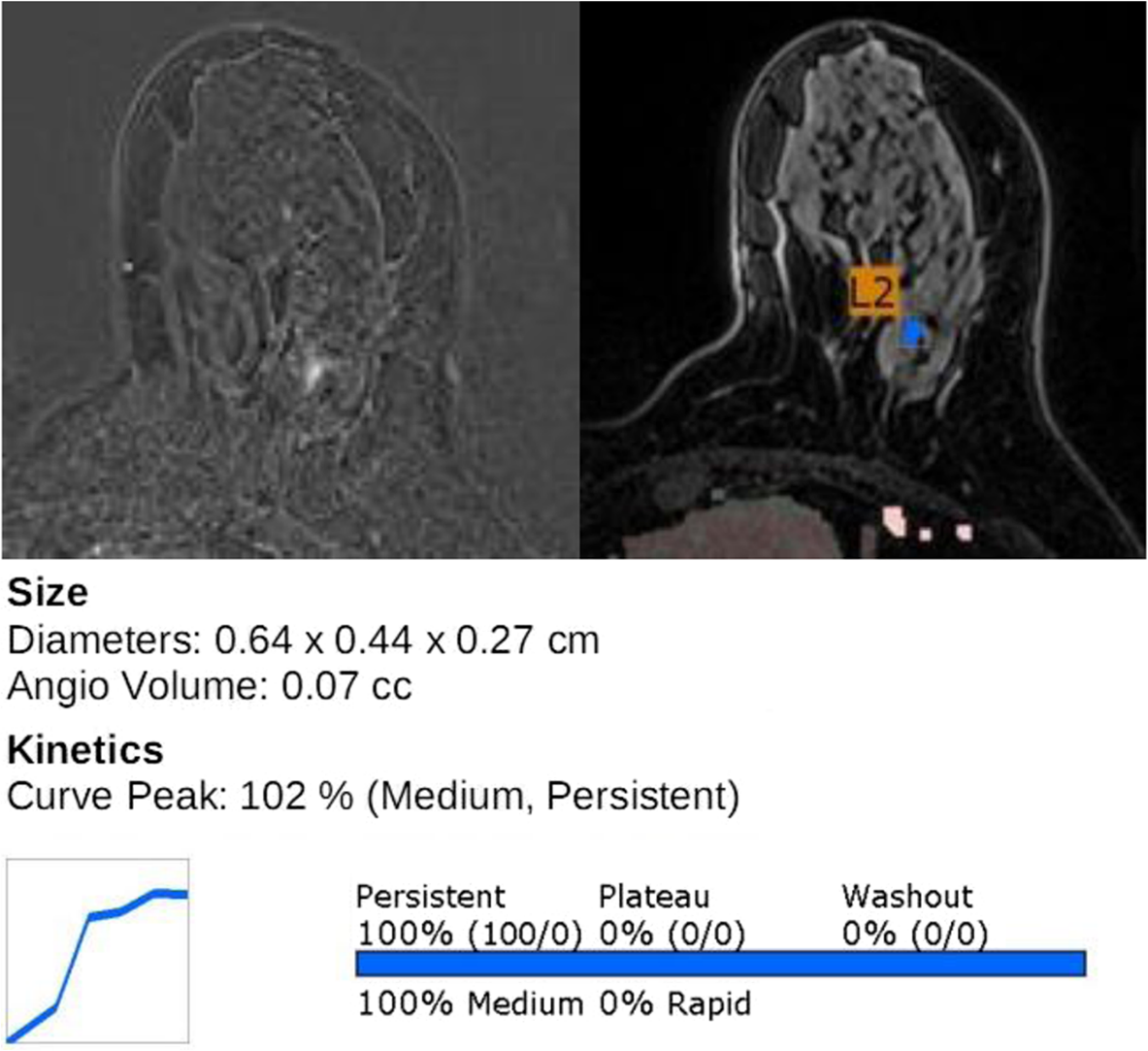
Imaging features of DCIS on final surgical pathology. Axial fat-suppressed T1-weighted contrast-enhanced MRI revealed a small oval-shaped mass with homogeneous enhancement (left). MRI with a CAD color overlay map revealed the tumor size and enhancement kinetics, i.e., a 102% peak enhancement and a 100% persistent component (right)
